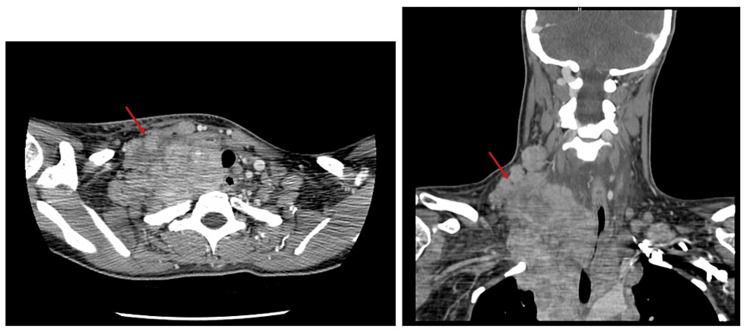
Computed tomography of the neck with contrast: ill-defined soft tissue mass (red arrows) diffusely involving the upper mediastinum, right supraclavicular fossa, and right inferolateral neck.
